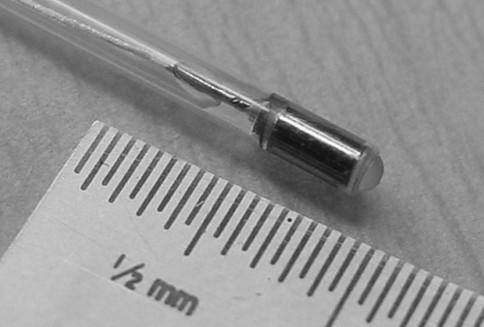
Exhale Doppler probe tip.
